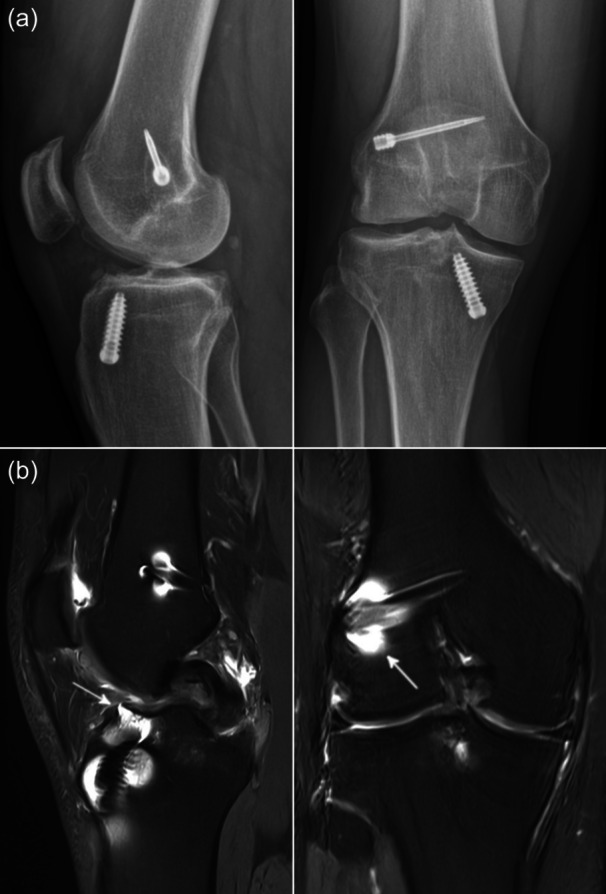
Unintended perturbations in image recognition. Anteroposterior and lateral radiographs (a) and proton density weighted coronal and sagittal magnetic resonance imaging (MRI) scans (b) of a right knee with failed transtibial anterior cruciate ligament reconstruction. Note the tibial and femoral implants for graft fixation and the corresponding metallic MRI artifacts (arrows).
